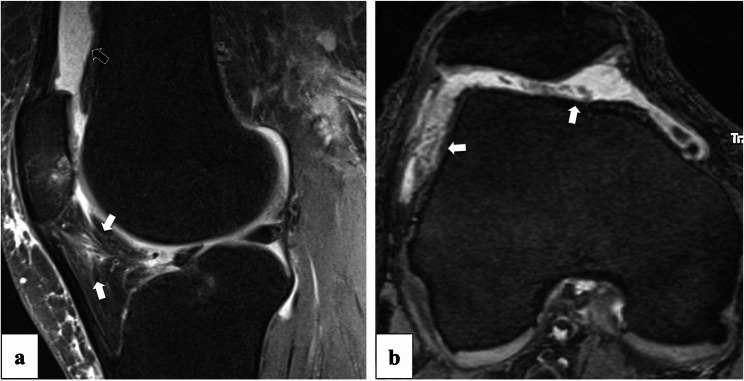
Image examples for an inflammatory phenotype. a = sagittal knee MRI with Hoffa synovitis (white arrows; Grade 2) and effusions synovitis (black arrow, Grade 3). b = axial knee MRI with moderate effusion synovitis (Grade 2) and extensive synovial proliferation (white arrows, Grade 3)
